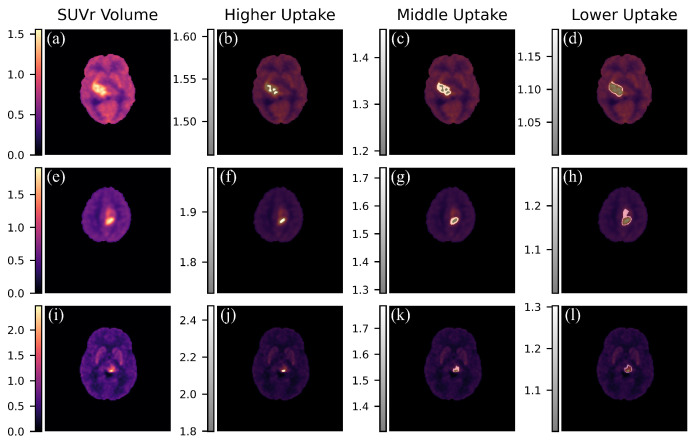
Visualization of tumor sub-volumes. (a–d): A Patient with a DMG H3 K27-altered (WHO grade 4) of the right thalamus. The first column depicts the [^18^F]F-DOPA PET SUVr volume. The following columns show the three sub-tumor masks. (e–h): A subject with a diffuse pediatric-type high-grade glioma, H3-wildtype, and IDH-wildtype (WHO grade 4) of the left paracentral lobule; in the following columns, we show the SUVr volume and the three masks. (i–l): Finally, we report a patient with a DMG H3 K27-altered (WHO grade 4) of the left thalamus, as shown in (i).
